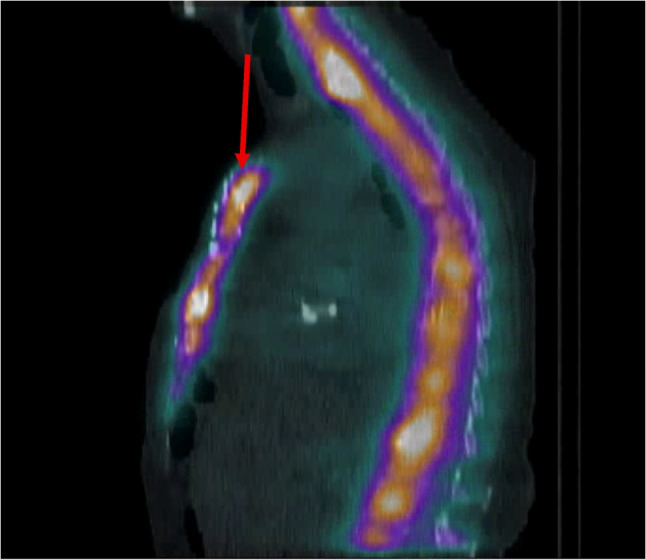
Sagittal SPECT CT image demonstrating high signal within the sternum
